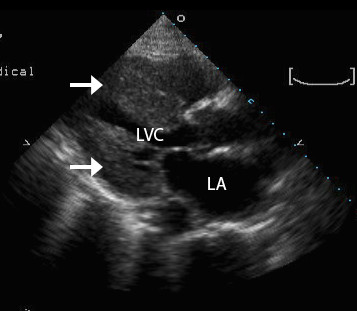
An Echocardiogram shows dramatic left ventricular hypertrophy (arrows) and a small ventricular cavity. LA - Left Atrium; LVC - Left Ventricular Cavity.
